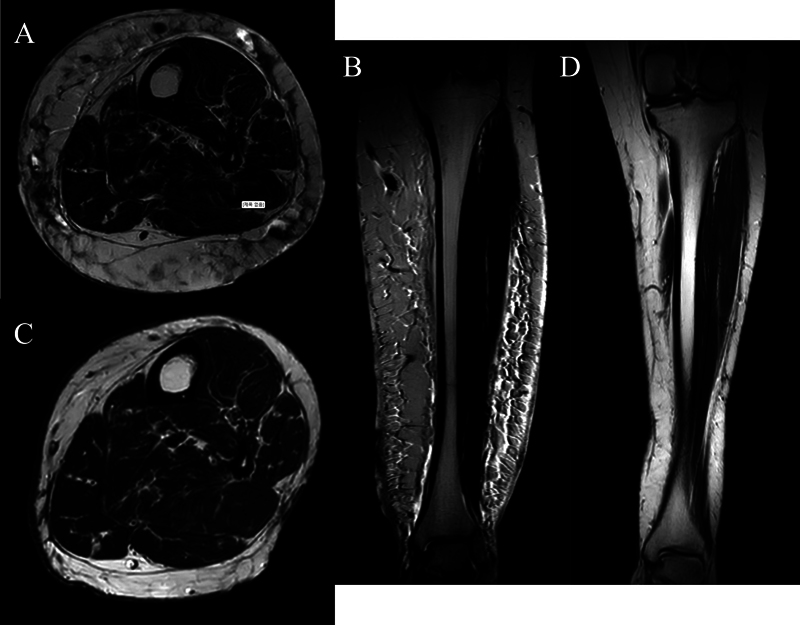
Magnetic resonance imaging assessment. Preoperative imaging workup with axial (
